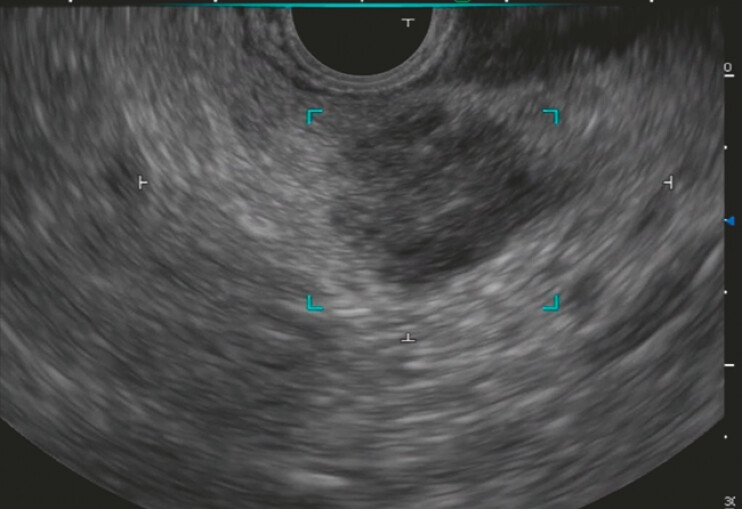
Using the EUS-AI system, the 15 mm lesion in the pancreatic tail previously detected via MRI was enclosed by the blue box. EUS-AI, EUS system assisted by artificial intelligence; MRI, magnetic resonance imaging.
